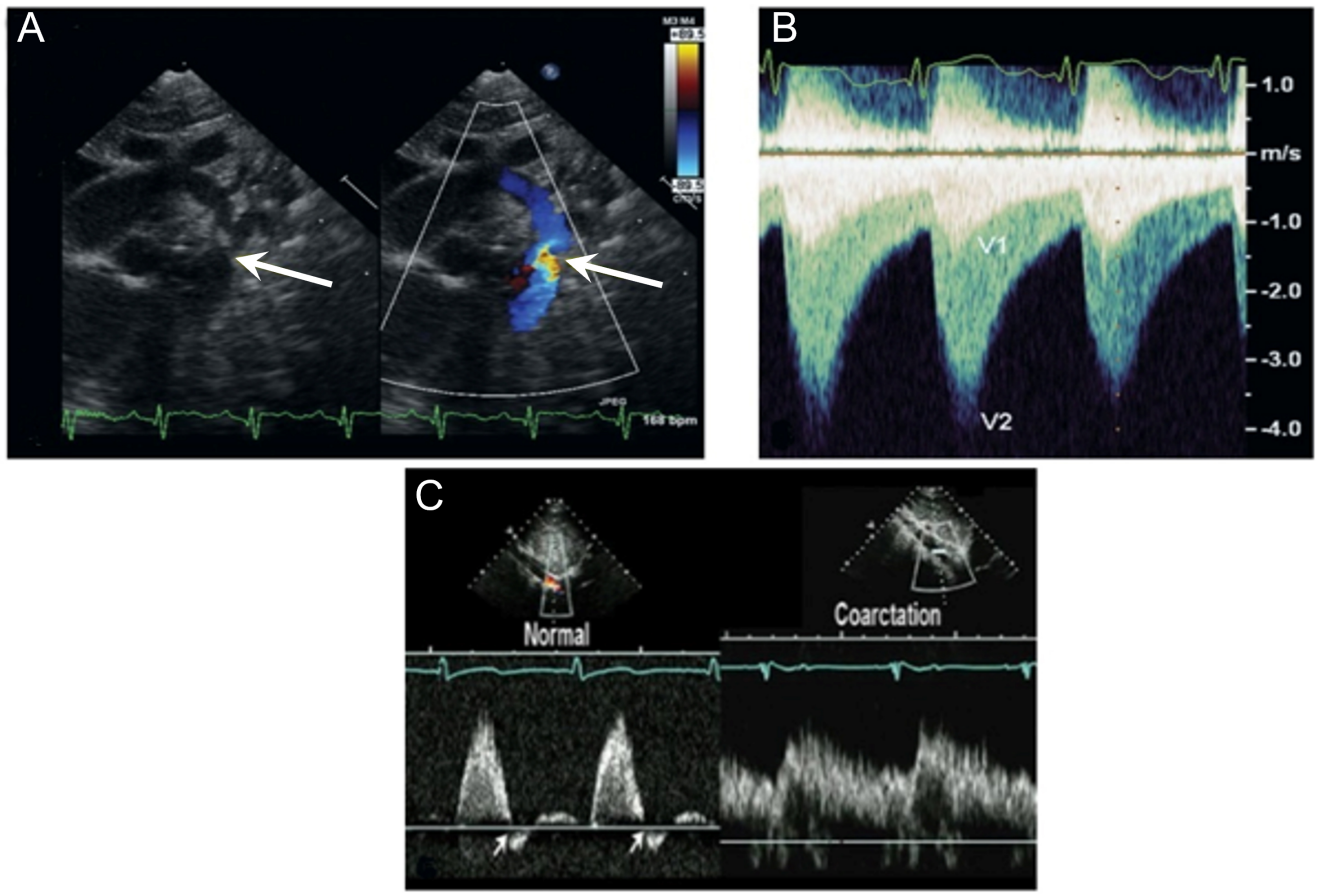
Echocardiographic evaluation of coarctation of the aorta.
